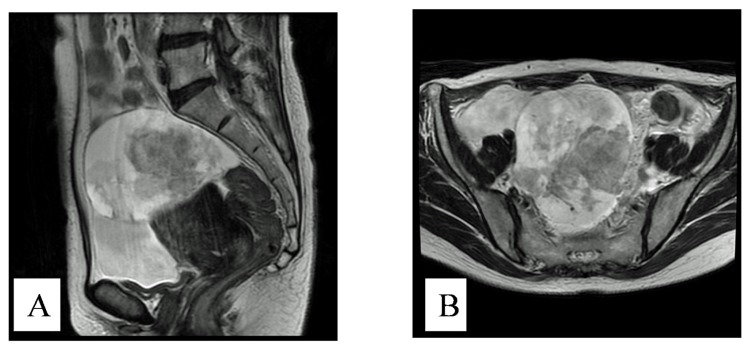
Enhanced magnetic resonance imaging (MRI) showing an irregular right adnexal mass, ascites. (A) Preoperative sagittal and T2-weighted images; (B) Preoperative axial and T2-weighted images.
